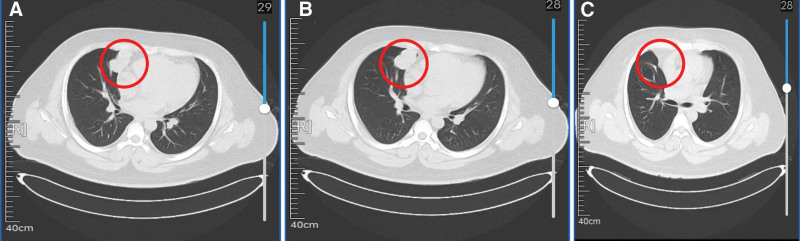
CT scan images during treatment. (A) CT scan upon admission (November 9, 2022); (B) The enhanced CT scan before the surgery (November 9, 2022); (C) CT reexamination 3 months after the surgery (March 23, 2023). CT = computed tomography.
